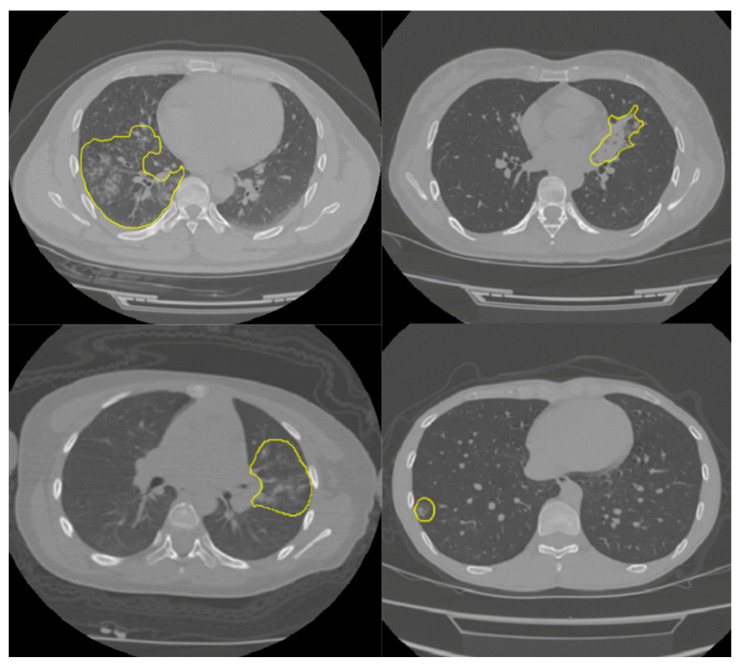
CT images showing the parenchyma with visible subregions (yellow) infected with COVID-19 disease.
